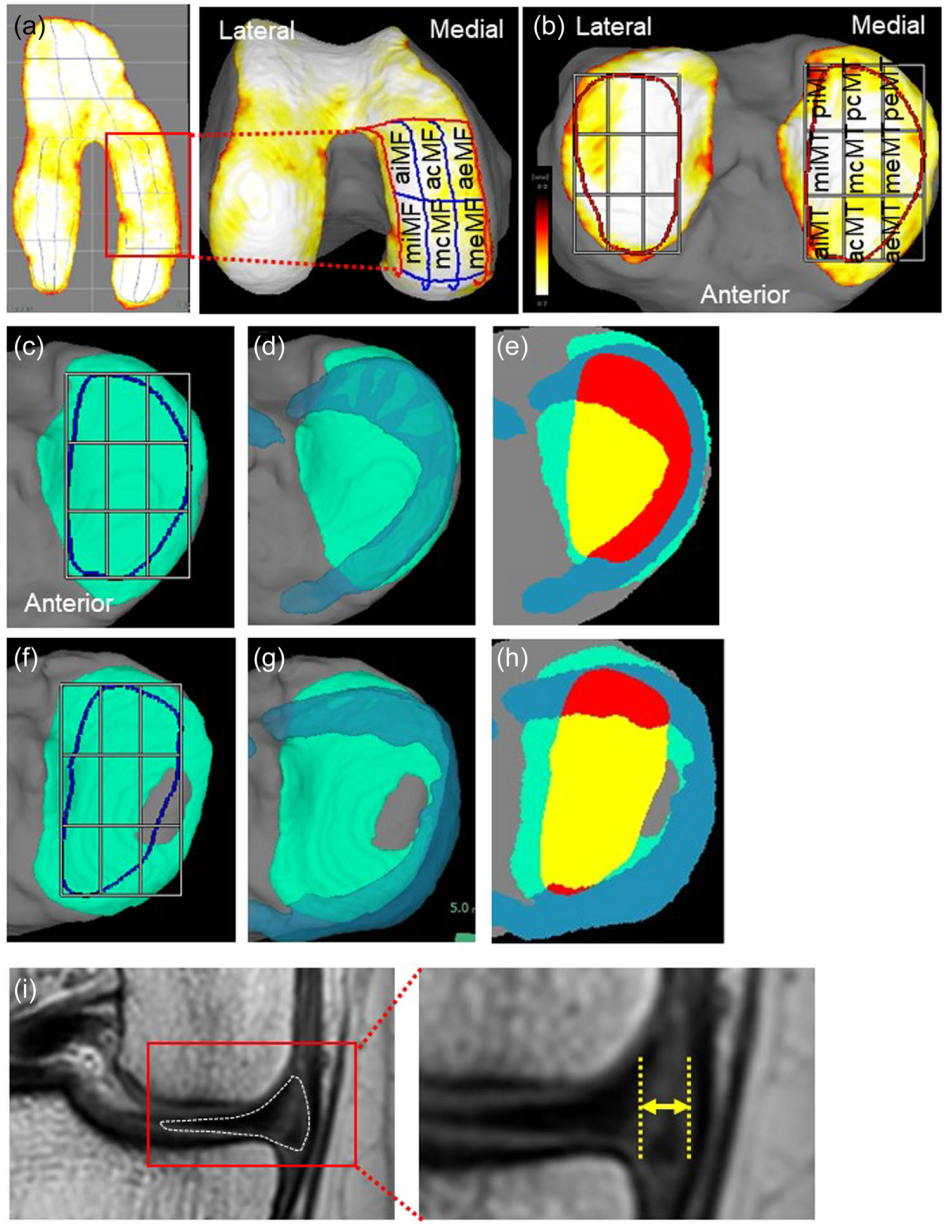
Analysis of the medial meniscus and cartilage of the medial femoral condyle and the medial tibial plateau in three‐dimensional (3D) and two‐dimensional (2D) magnetic resonance imaging (MRI). (a) Radially (left) and vertically (right) projected cartilage thickness mapping and subregions of the medial femoral cartilage. acMF, anterior central MF; aeMF, anterior external MF; aiMF, anterior internal MF; MF, medial femur; mcMF, middle central MF; meMF, middle external MF; miMF, middle internal MF. (b) Vertically projected cartilage thickness mapping and subregions of the tibial cartilage. acMT, anterior central MT; aeMT, anterior external MT; aiMT, anterior internal MT; mcMT, middle central MT; meMT, middle external MT; miMT, middle internal MT; MT, medial tibia; pcMT, posterior central MT; peMT, posterior external MT; piMT, posterior internal MT. (c) Medial compartment of the tibia in a healthy knee. The cartilage is coloured in green, and the region of interest (ROI) for the cartilage is represented in blue lines. (d) Medial tibial cartilage with the medial meniscus (MM) in a healthy knee. MM is shown in light blue. (e) Medial meniscus covering ratio (MMCR) in a healthy knee. The portion of the MM within the cartilage ROI is indicated in red, while the portion outside the ROI is shown in blue. The area of the cartilage not covered by the MM within the ROI is marked in yellow. MMCR refers to the ratio of the red area to the sum of the yellow and red areas. (f) The medial compartment of the tibia in a case involving medial meniscus extrusion (MME). The tibial cartilage is indicated in green, while the ROI is shown in blue lines. (g) Medial tibial cartilage and the MM in a case of MME. MM is shown in light blue. (h) MMCR in the case of MME. (i) MME width analysed by 2D MRI coronal plane. The left panel shows the medial compartment of the knee. The right panel shows a high‐magnification image of the MM. The white dotted line represents the MM, and the yellow arrow indicates MME width.
